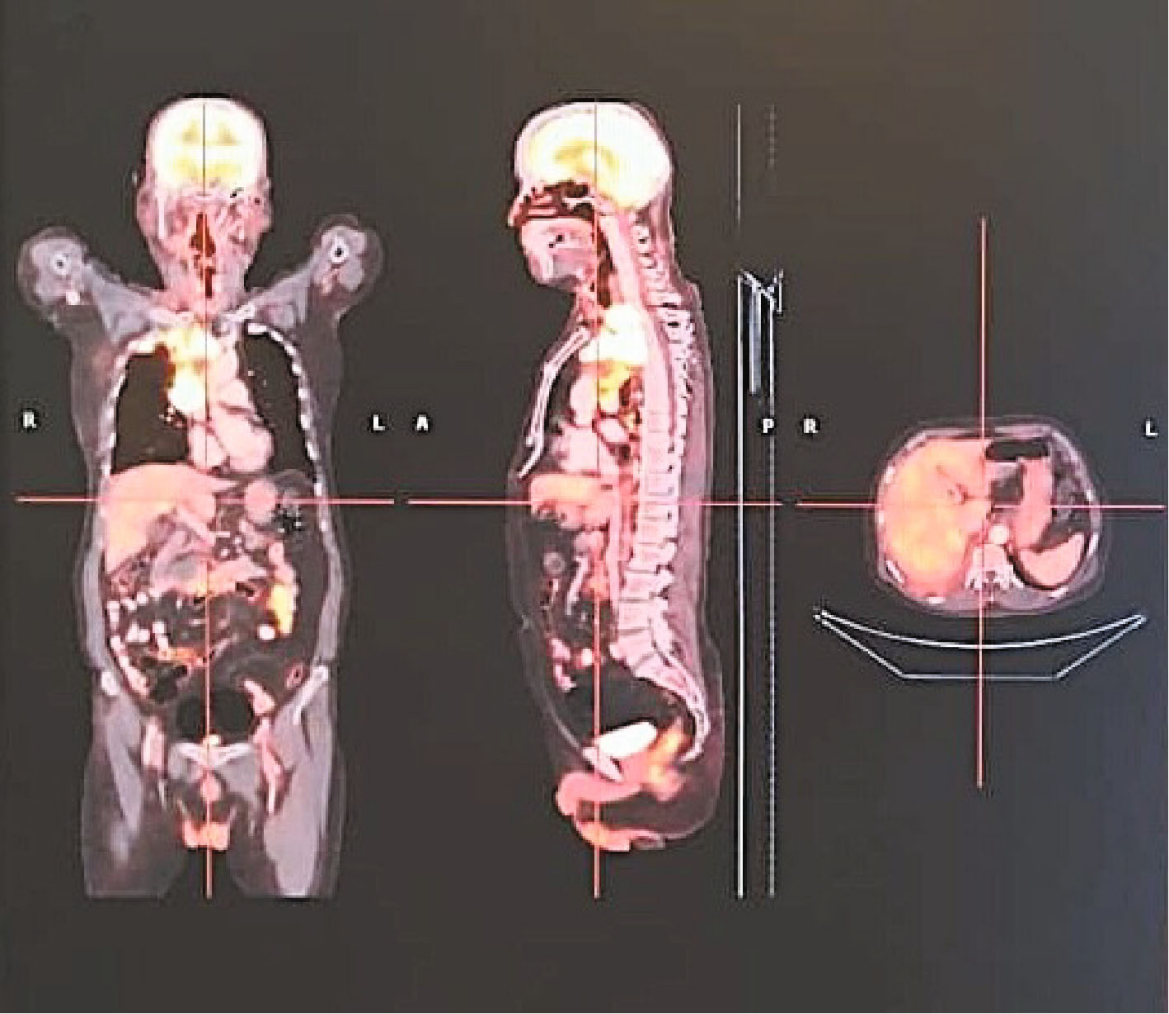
PET-CT showing a large mass in the right upper lobe of the lung, with associated mediastinal lymphadenopathy and right-sided pleural effusion.
